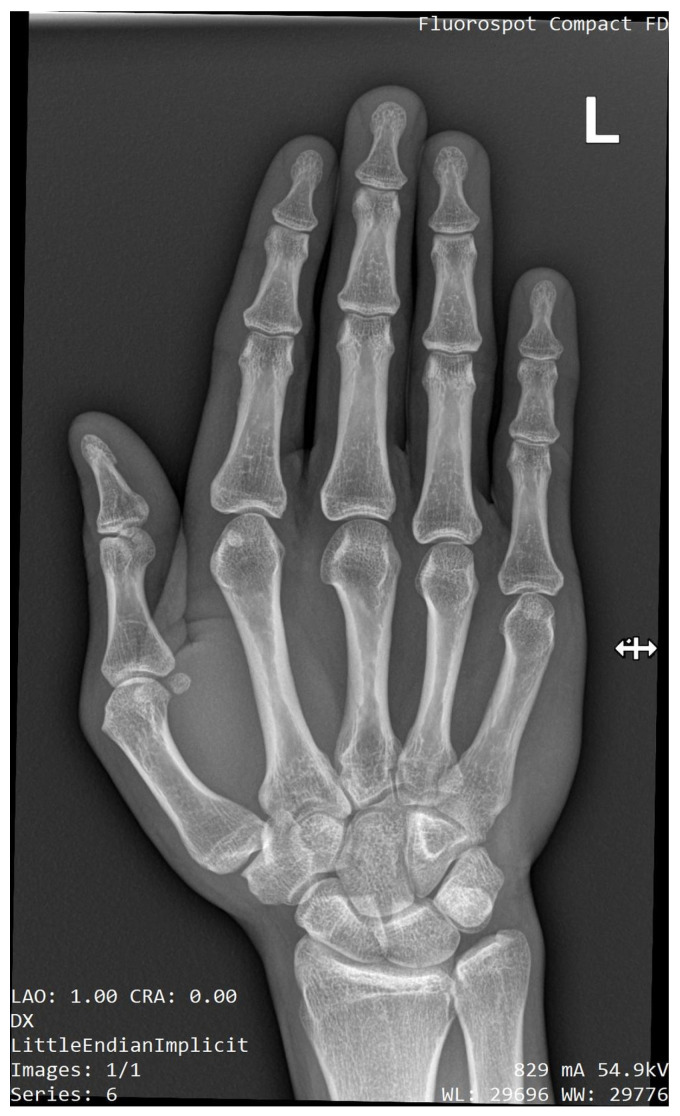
Radiographic image of the wrist joint in antero-posterior view showing hypoplasia of the ulnar styloid process.
